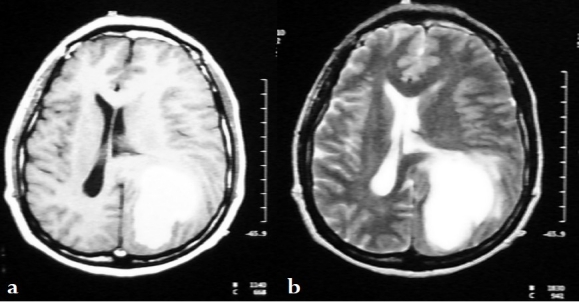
(a, b) Cranial MRI with gadolinium revealing a 6 × 5 × 4 cm left-sided parieto-occipital lesion with brain edema, associated mass effect and uncal herniation. The lesion was composed of cystic tissue separated by various fibrous septae. The tumor was hyperintense on T1-(a) and T2-(b) weighted images and showed heterogeneous enhancement with contrast peripherally.
